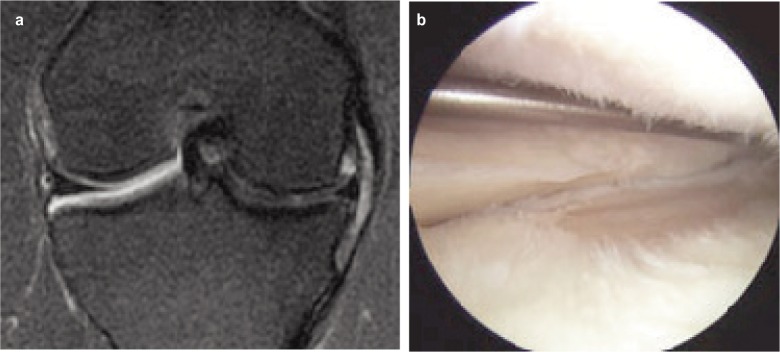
Meniscal extrusion on MRI and the arthroscopic image of the tear.
